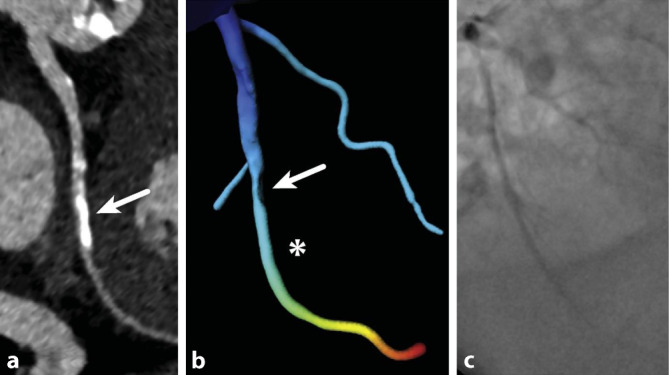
Neben dem klinisch etablierten Agatston-Score werden auch quantitative funktionelle Parameter beforscht. Hier gibt es vielversprechende Ergebnisse zur CT-basierten fraktionellen Flussreserve (CT-FFR; Abb. 3; [19]) – dem Pendant zur mittels invasiver Druckmessung im Herzkatheter bestimmten FFR [27]. Die FFR gibt das Verhältnis des mittleren arteriellen Drucks hinter einer Koronarstenose im Vergleich zum maximalen Druck vor der Stenose unter Adenosinbelastung an und wird mittlerweile als Goldstandard zur Ermittlung der hämodynamischen Relevanz einer Koronarstenose angesehen [23].
